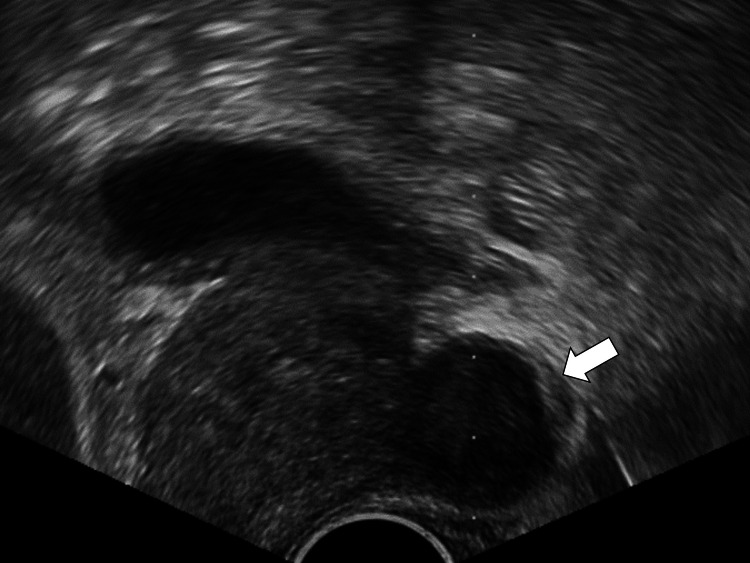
Image of ultrasound-guided fluid aspiration from the prostate.
